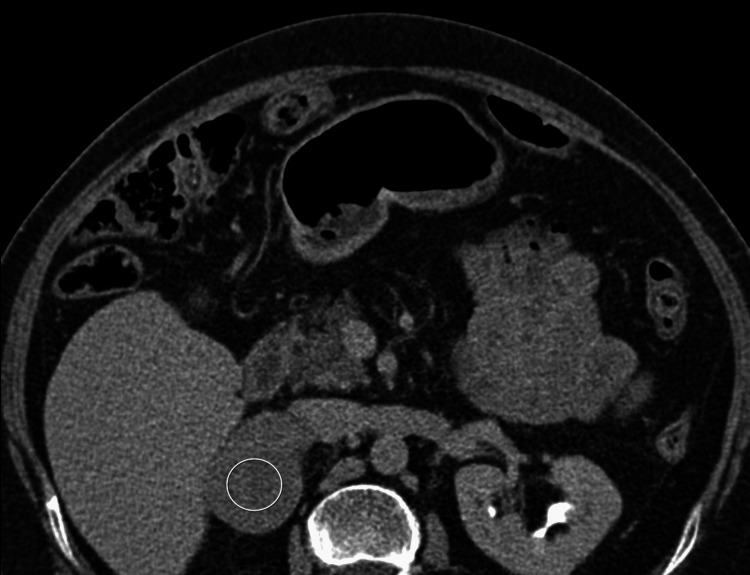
Computed tomography (CT) scan of the abdomen demonstrating incidentally noted adrenal mass. *White circle*: Large irregular right-sided adrenal mass with foci of heterogenous internal enhancement noted
